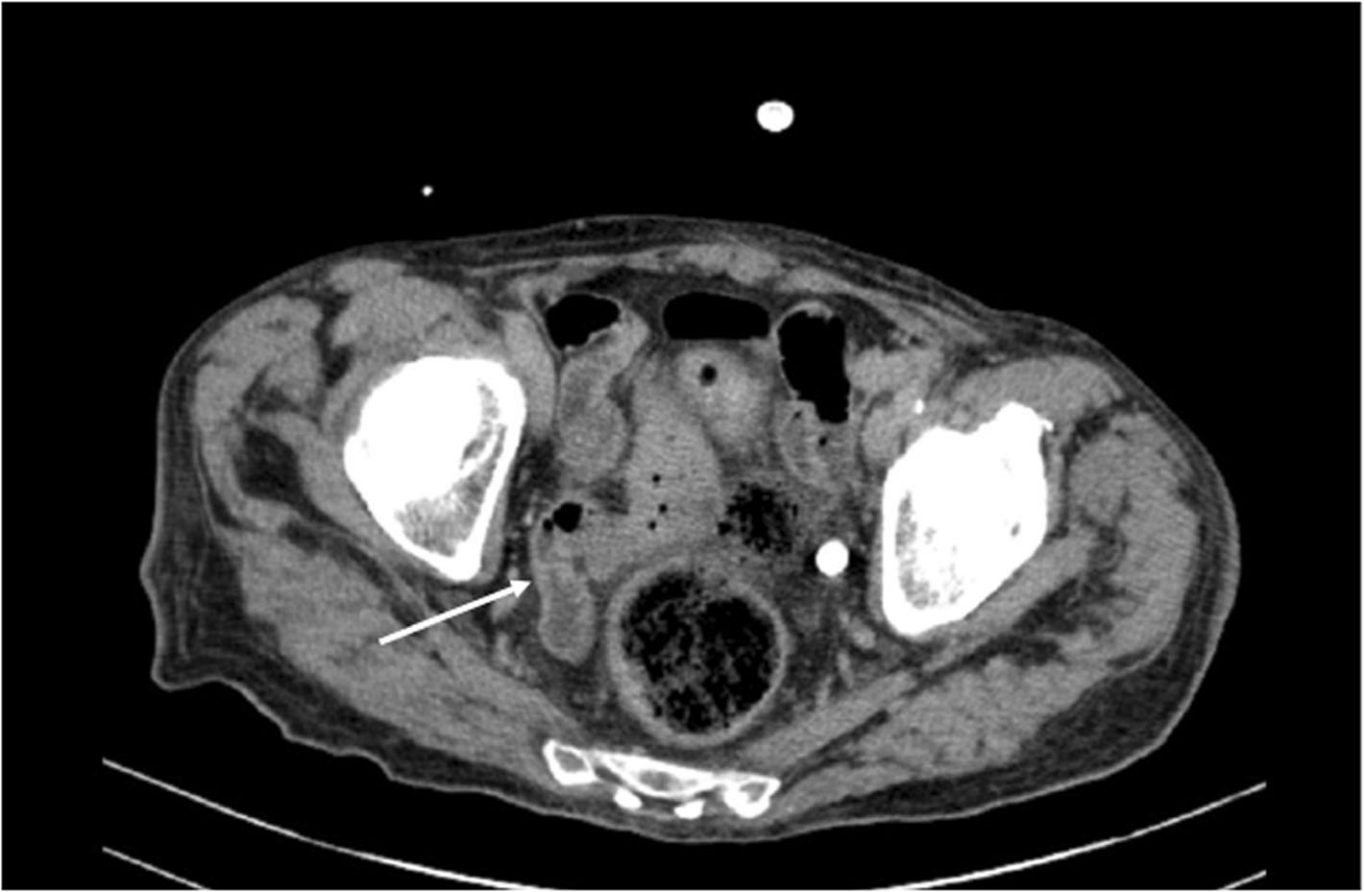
Abdominal CT scan. The arrow shows parietal thickening of the ileo-caecal region
